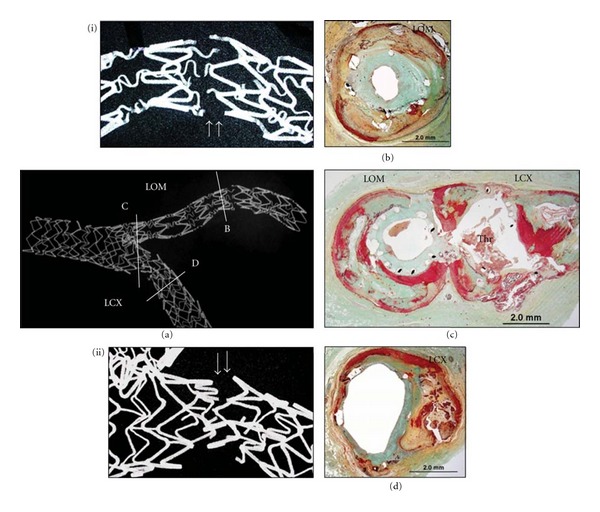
A case of grade V stent fracture: SES and PES. A 68 year-old woman died suddenly from stent thrombosis in left circumflex artery (LCX) 172 days following stent implantation. Radiograph showing the stented LCX and left obtuse marginal artery (LOM) (a). Note, presence of grade V sirolimus-eluting stent (SES) fracture highlighted in magnified image (i) and another grade V fracture at the bifurcation site in the paclitaxel-eluting stent (PES) (ii). SES in LOM with grade V fracture was associated with restenosis (b). PES fracture in LCX was located in the area close to the bifurcation site where the thrombus was located (Thr). The stented LCX segment distal to the fracture was widely patent (d). (Reproduced with permission from [46].)
